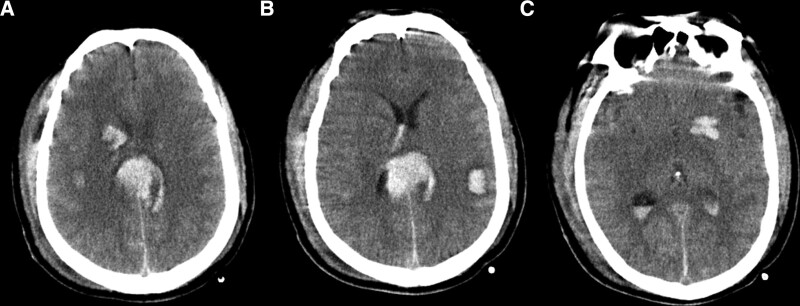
Preoperative cranial CT. CT = computed tomography.
